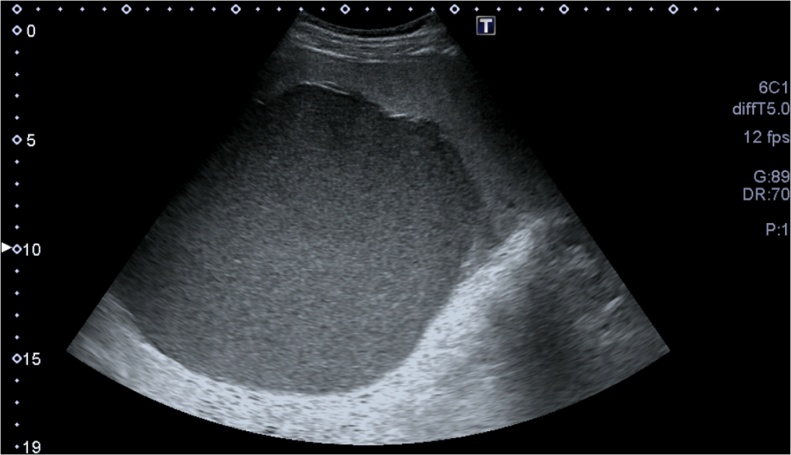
Abdominal ultrasound showing a large splenic cyst containing homogenous internal echoes/debris.
